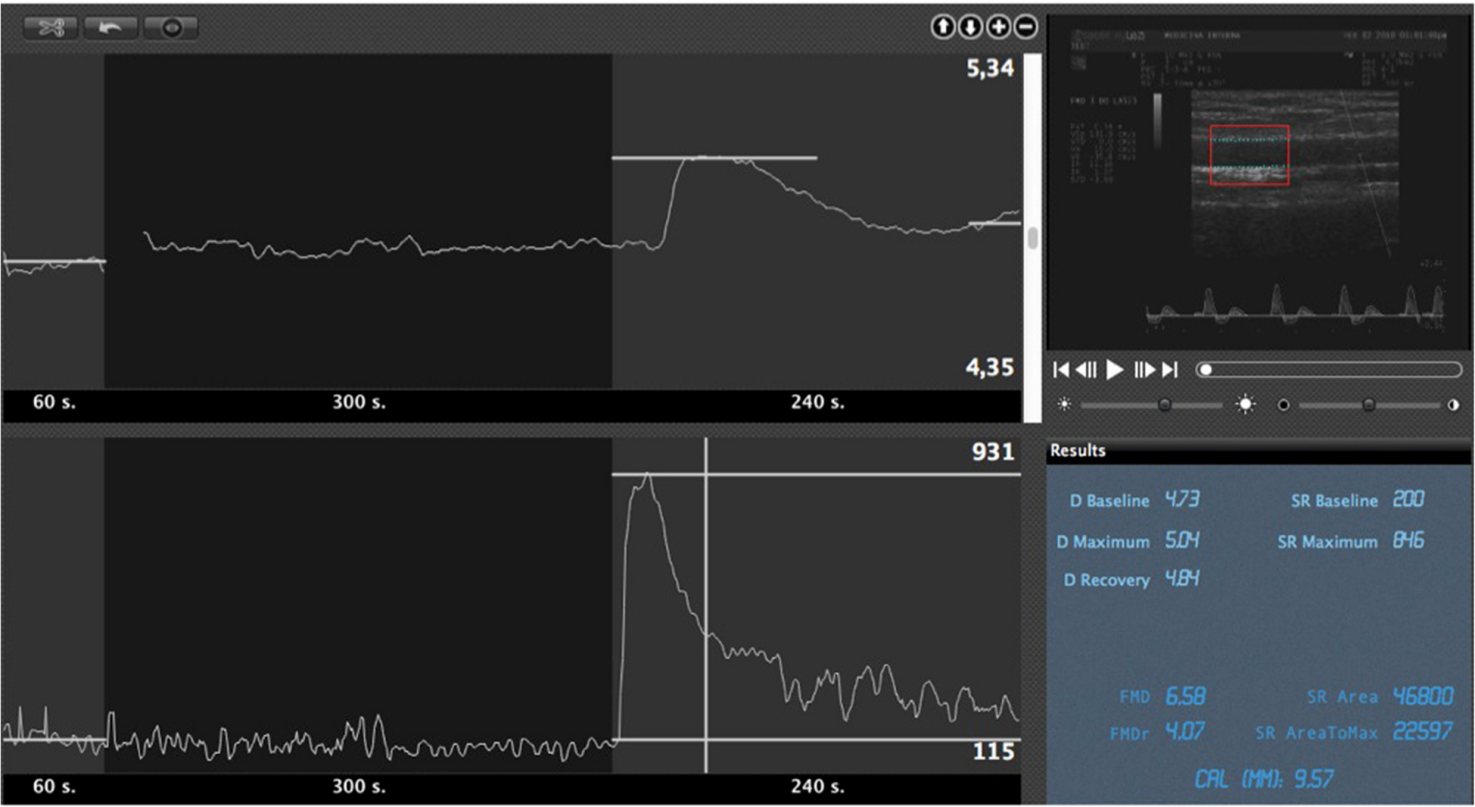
Example of automatic edge detection and real-time analysis of B-mode and doppler signal during flow mediated dilation (FMD) assessment (top right). Graphs show changes in brachial artery diameter diameter (D, top panel) and shear rate (SR, left). The panel on bottom left reports mean baseline, maximun) values of D and SR, and the computation of FMD.
